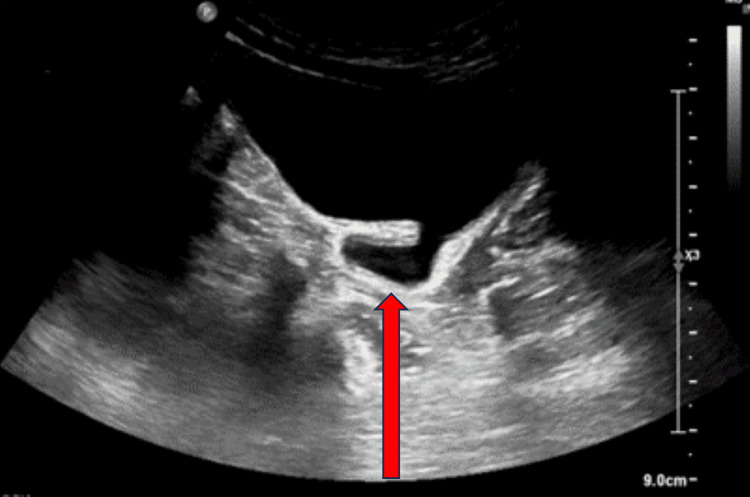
Ultrasound image of the urinary bladder showing the two compartments of the hourglass urinary bladder
